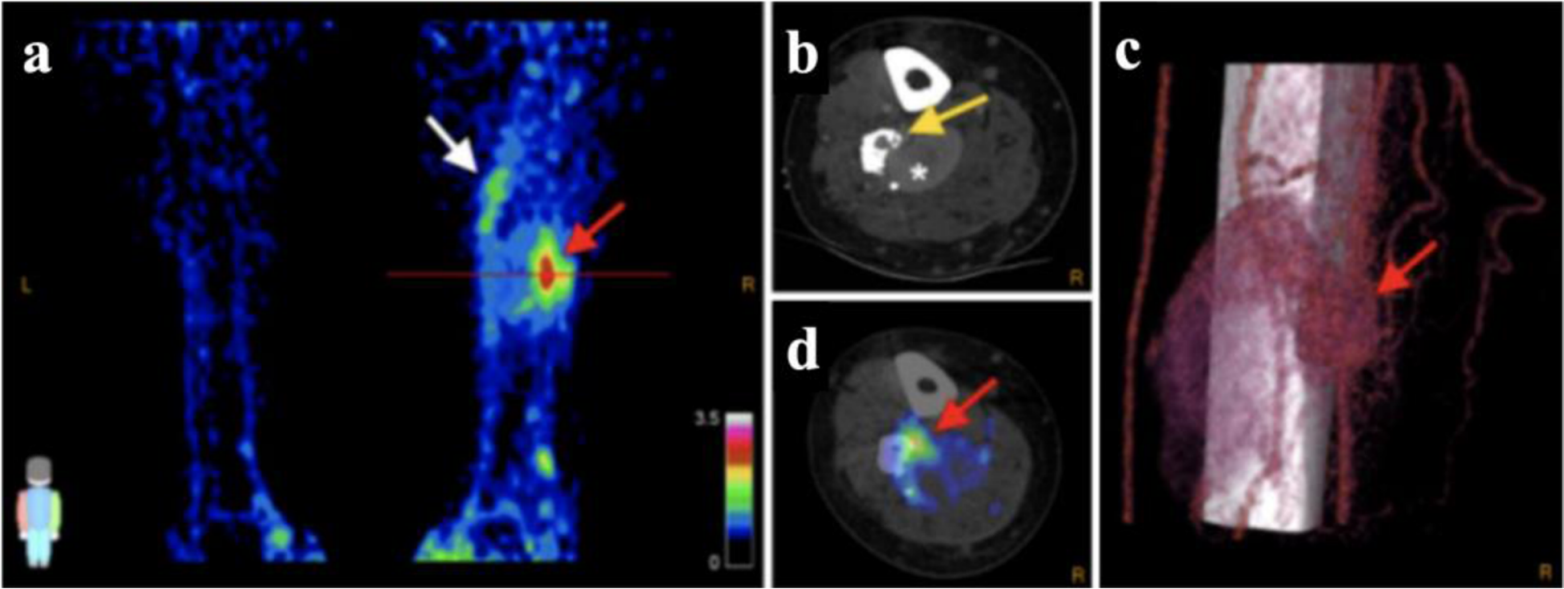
^68^Ga-RGB PET/CT in 38-year-old man with right-leg AVM. Maximal-intensity ^68^Ga-RGD PET projection (a), CTA (b) and 68Ga-RGD PET/CT (d), and 3D reconstruction of 4D-CTA (c) show nidus (SUV_ma*x*_ 3.2; SUV_peak_ 2.5, red arrows). Axial plane in (b) and (d) is at location of line in (a). Heterogeneous pattern of enhanced uptake is seen in tissue adjacent to nidus at more proximal part of the right leg (white arrow); bone deformation in the fibula caused by compression and infiltration of vessels is also seen (yellow arrow), along with large venous aneurysm (asterisk). Arterial flow with nidus and fistula is seen in (c), along with upcoming venous flow and the large venous aneurysm. This research was originally published in JNM. Lobeek D, Bouwman FCM, Aarntzen EHJG, Molkenboer-Kuenen JDM, Flucke UE, Nguyen HL, Vikkula M, Boon LM, Klein W, Laverman P, Oyen WJG, Boerman OC, Terry SYA, Schultze Kool LJ, Rijpkema M. A Clinical Feasibility Study to Image Angiogenesis in Patients with Arteriovenous Malformations Using ^68^Ga-RGD PET/CT. J Nucl Med 2020; 61:270–275.© SNMMI
